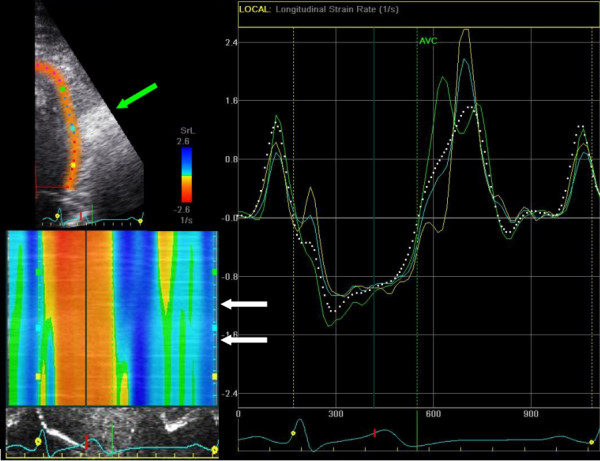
Impact of stationary artifact on 2DSE. 2DSE derived strain-rate of one cardiac cycle in the lateral wall in the same ultrasound examination of figure 6. *Top left*: B-mode recording with 2DSE ROI of the lateral wall. *Bottom left*: Color-coded M-mode of strain-rate values. The stationary artifact can be seen at the level of the green arrow. Due to the implementation of a clutter filter in the tracking algorithm, 2DSE is less influenced by artifacts. Note the normal SR curves (*right*) despite the prominent artifact. Consequently, no reversal of strain-rate is seen in the color-coded M-mode between the white arrows (compare to figure 6). The appropriate ROI tracking can be seen in the additional file (see Additional File 2)
